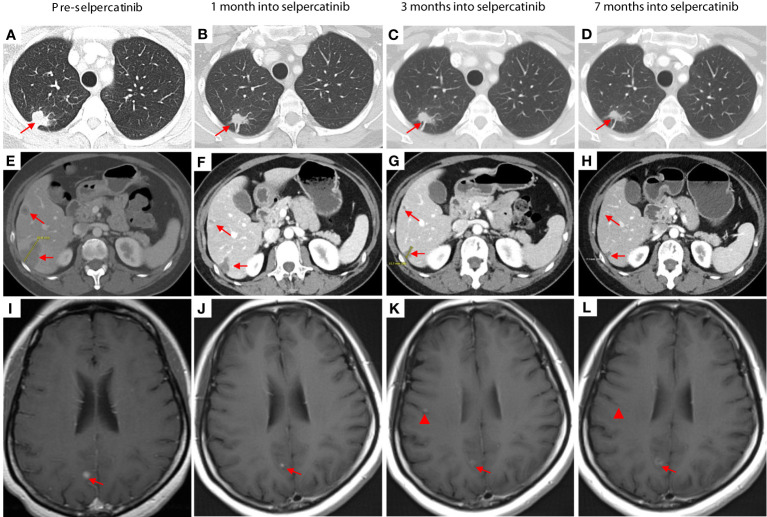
Pretreatment images were obtained 3 weeks prior to the initiation of chemotherapy. The images in the second column were taken 1 month after the start of selpercatinib treatment (3 weeks after the first day of the second cycle of chemotherapy). CT images show shrinkage of lesions in the right upper lobe (A–D) and in the liver (E–H). Brain lesions responded to selpercatinib [(I–L), arrows] except for the development of tiny new brain lesions when the patient was on a low dose of selpercatinib 40 mg twice daily [(K), arrowhead], which have improved [(L), arrowhead] or remained stable with subsequent increases in dose of selpercatinib. At 7 months, the patient was receiving a dose of 120 mg of selpercatinib in the morning and 80 mg in the afternoon.
